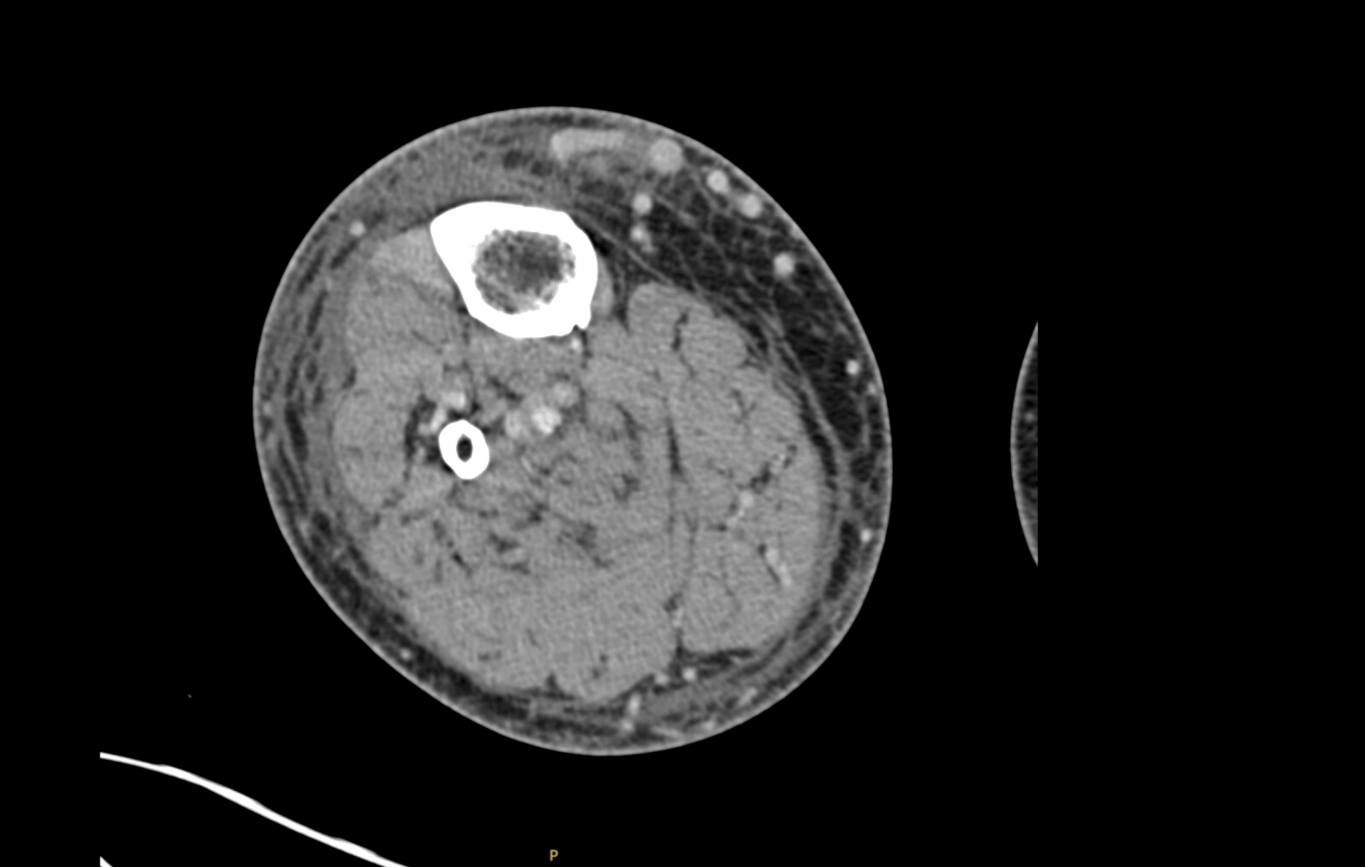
Lower extremity CT with contrast of the right lower extremity showing lower soft tissue edema and cellulitis without drainable fluid collection and no findings of osteomyelitis.
